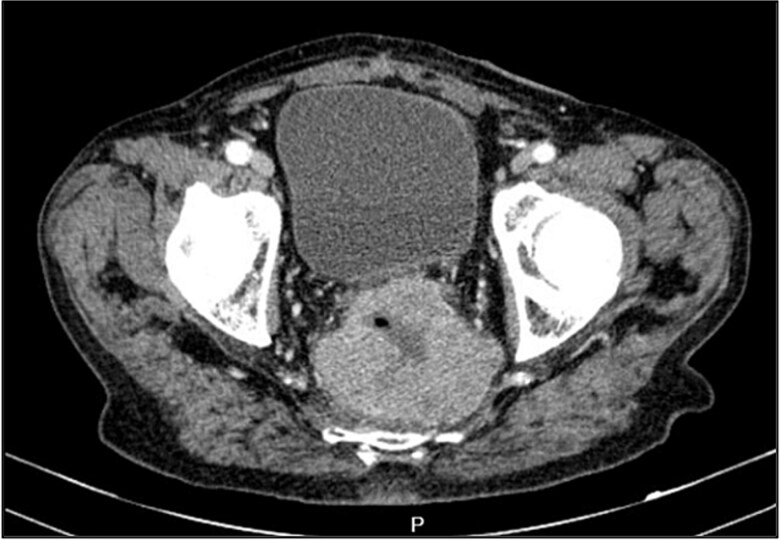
Pelvic recurrence (CT scan—Axial plane).
